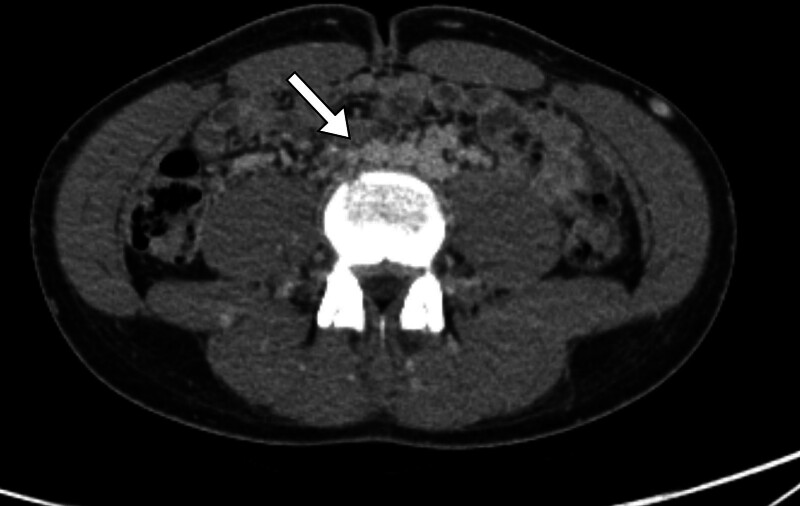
CT image of pre-anticoagulation. The absence of infrarenal IVC. CT = computed tomography, IVC = inferior vena cava.
